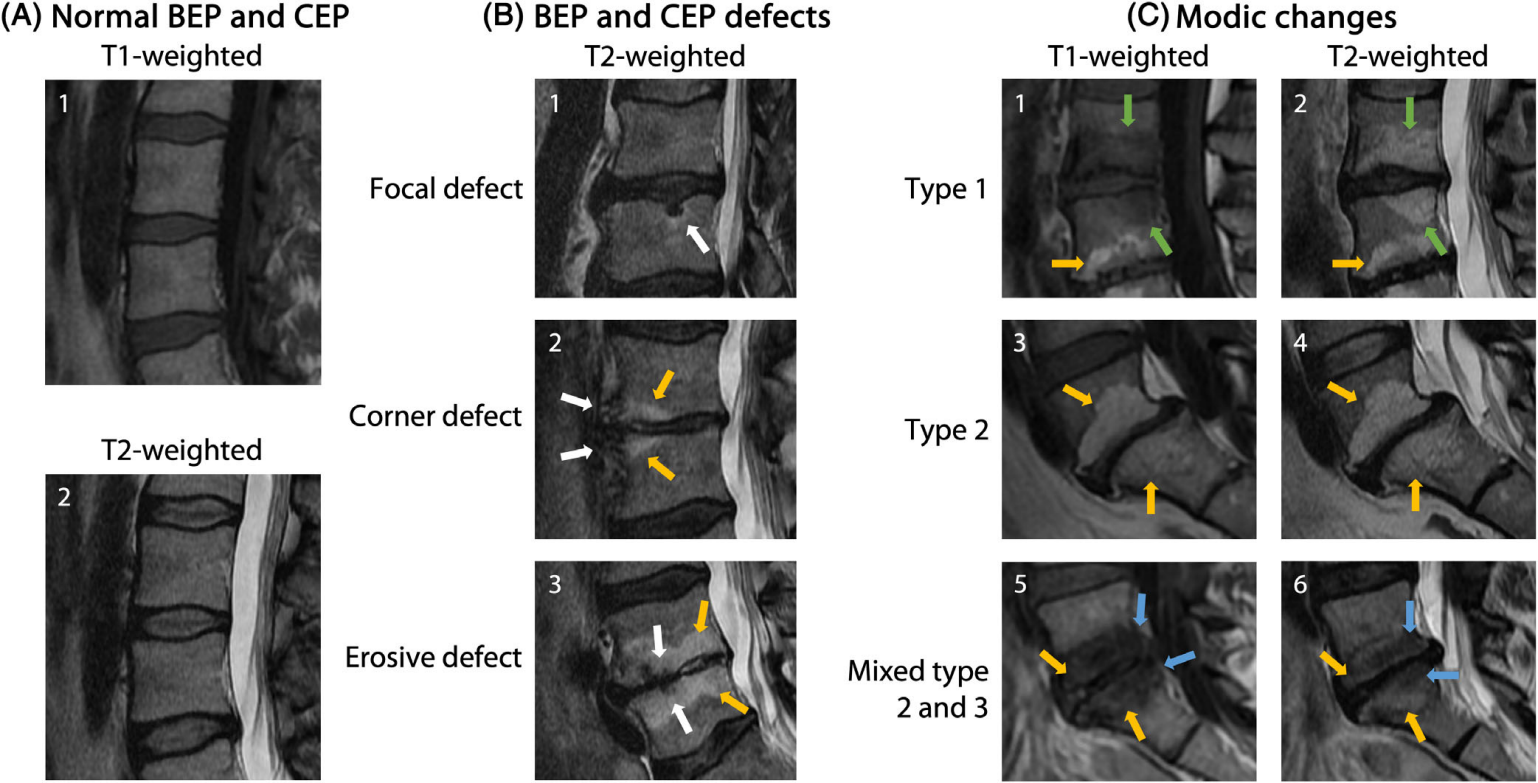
Examples of CEP and BEP appearance in standard of care MRI from 47‐year‐old participants in the Northern Finland Birth Cohort 1966. (A.1–A.2) Characteristic appearance of healthy CEP and BEP on T1‐ and T2‐weighted MRI. (B.1) Schmorl's node as an example of a focal defect at the L3–L4 IVD caudal BEP (white arrow); (B.2) corner defects at the anterior edges of the L4–L5 IVD cranial and caudal BEP (white arrows) with accompanying type 2 MC (orange arrows); (B.3) erosive defects at the L4–L5 IVD cranial and caudal BEP (white arrows) with accompanying type 2 MC (orange arrows); (C.1–C.2) type 1 MC extending from the L3–L4 IVD cranial and caudal BEP (green arrows) with type 2 MC also visible in the L4 vertebral body (orange arrows); (C.3–C.4) type 2 MC extending from the L5–S1 IVD cranial and caudal BEP (orange arrows); (C.5–C.6) type 2 and type 3 MC (orange and blue arrows, respectively) extending from the L5–S1 IVD cranial and caudal BEP.
